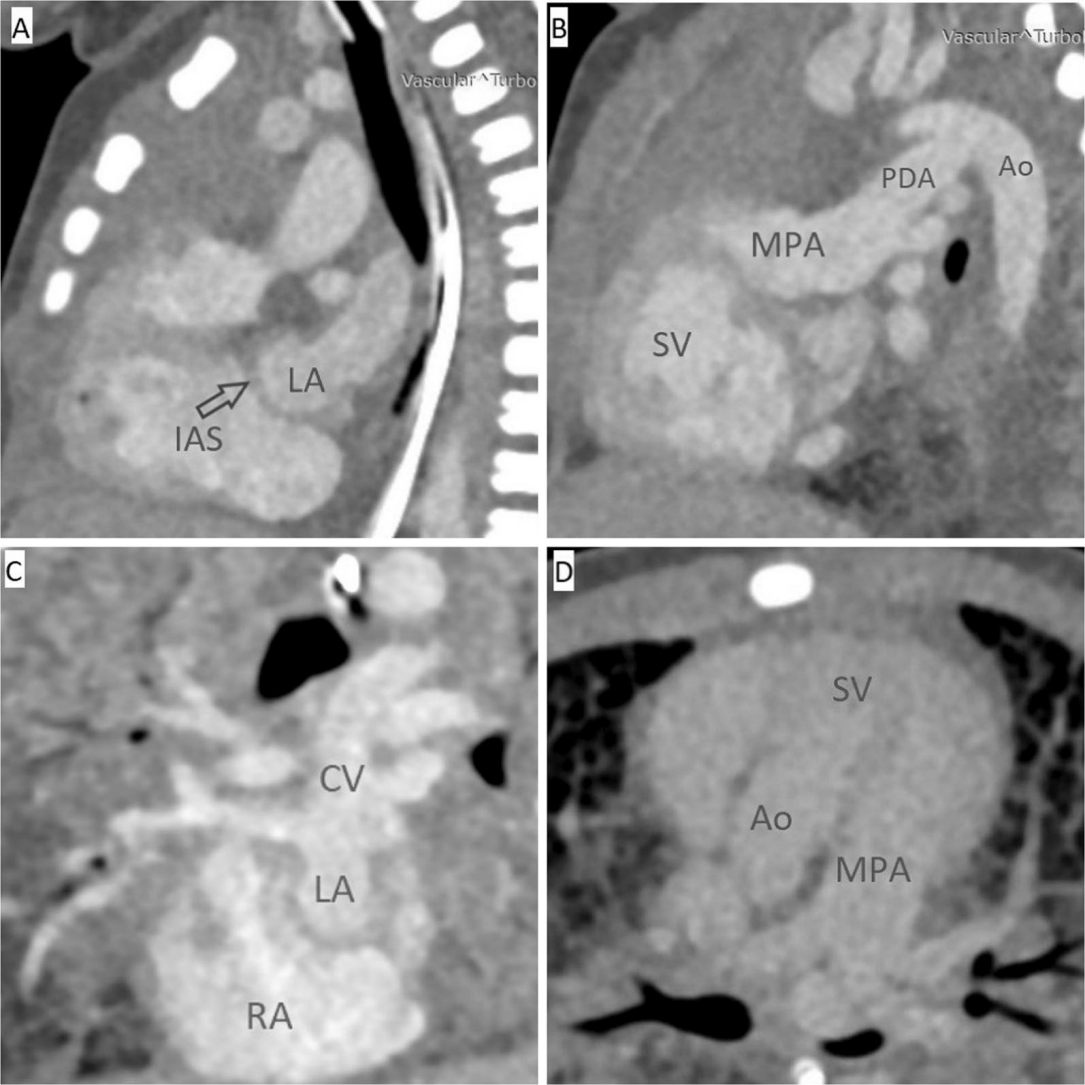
CT of chest. A Intact atrial septum (indicated by arrow). B Patent ductus arteriosus (indicated by arrow). C Cardinal vein (the only way outflow from the left atrium). D The aorta and pulmonary artery go from a single ventricle. *Ao* aorta, *CV* cardinal vein, *IAS* intact atrial septum, *LA* left atrium, *MPA* main pulmonary artery, *PDA* patent ductus arteriosus, *RA* right atrium, *SV* single ventricle
